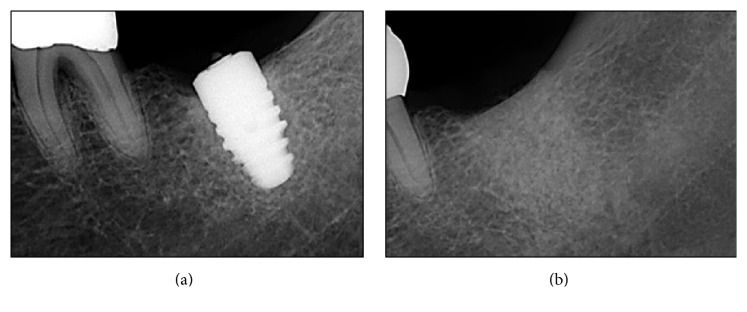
Postoperative radiographic view (periapical X-ray image). (a) Implant fixture with a fractured abutment screw. (b) Implant removal site filled with a bone grafting material.
